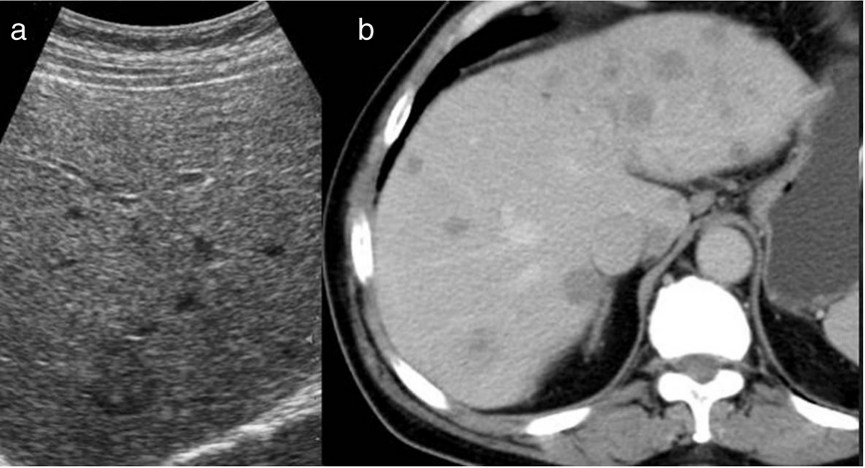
Radiological evaluation of liver metastases. (a) Ultrasound. (b) Unenhanced computed tomographic scan.
